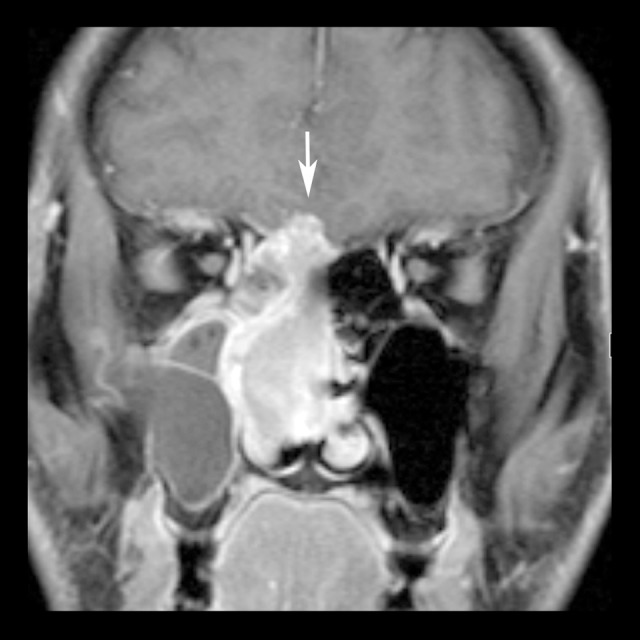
Olfactory neuroblastoma in a 38-year-old man. Coronal post-contrast, fat-suppressed, T1-weighted image shows homogenously enhancing tumor in the right nasal cavity with intracranial extension (arrow).
